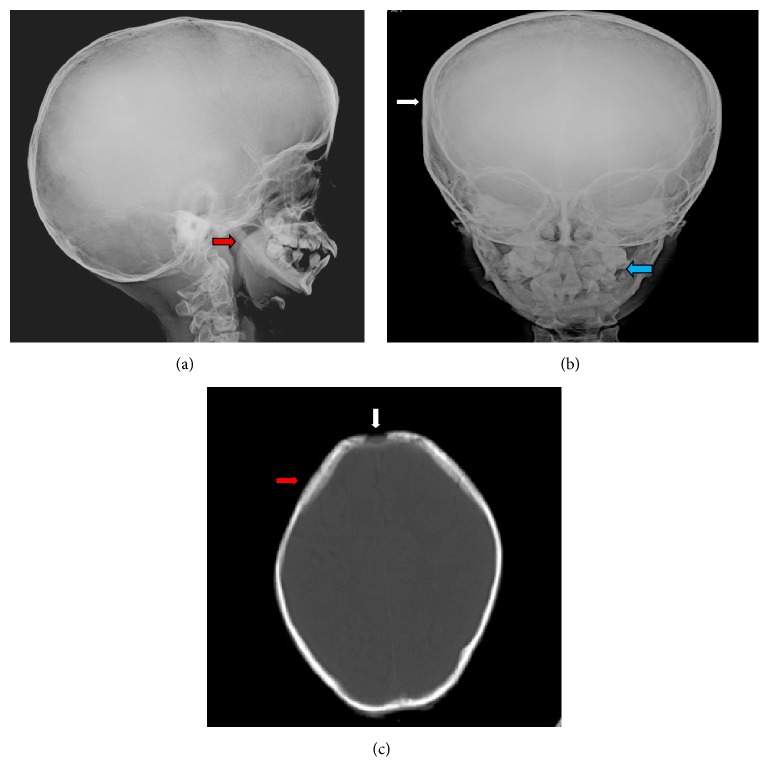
Skull radiograph lateral and anteroposterior views (a, b) showing disproportionate large calvarium with hypoplastic facial bones, small mandible with small ascending ramus, and obtuse mandibular angle (red arrow) and overcrowding of teeth (blue arrow). CT head (c) showing open anterior fontanelle (white arrow) and large cranial vault with thin diploic space (red arrow).
